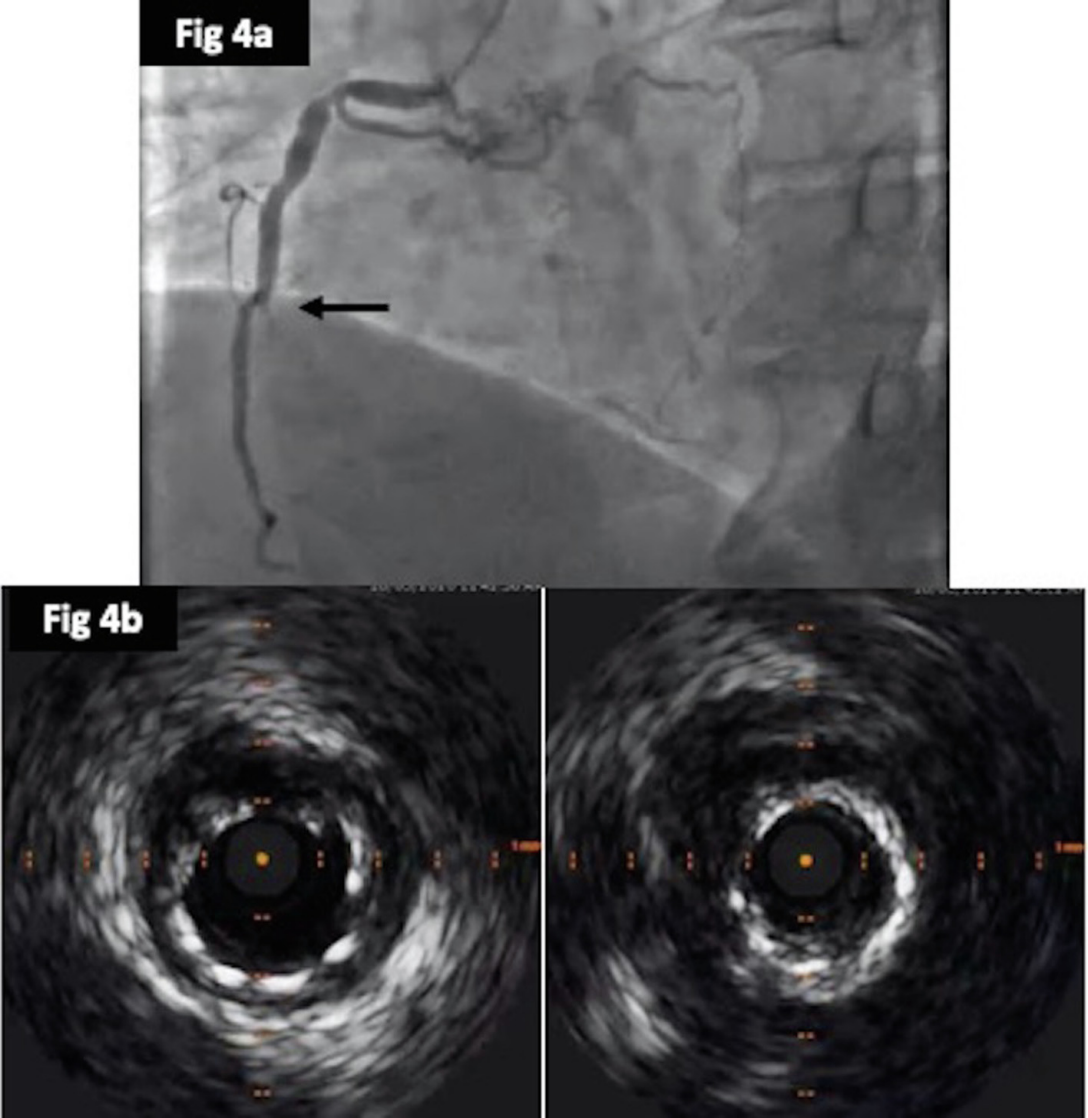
4a: Coronary angiogram showing occluded mid-segment of RCA within the previously stented segment 4b: Intra-vascular ultrasound (IVUS) exhibiting significantly under deployed stent.
